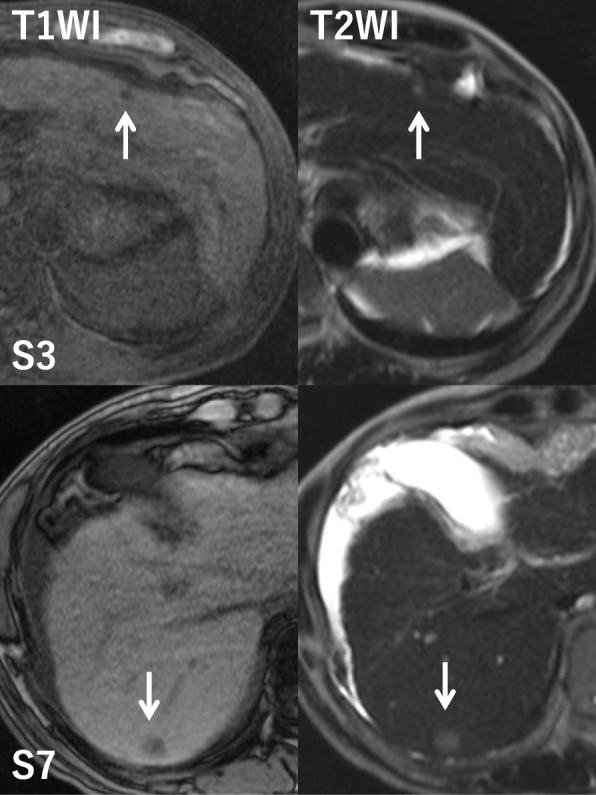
Abdominal magnetic resonance imaging. Magnetic resonance imaging revealed similar findings of low signal intensity on T1-weighted images and high signal intensity on T2-weighted images for both tumors (*arrows*). *T1WI* T1-weighted image, *T2WI* T2-weighted image
